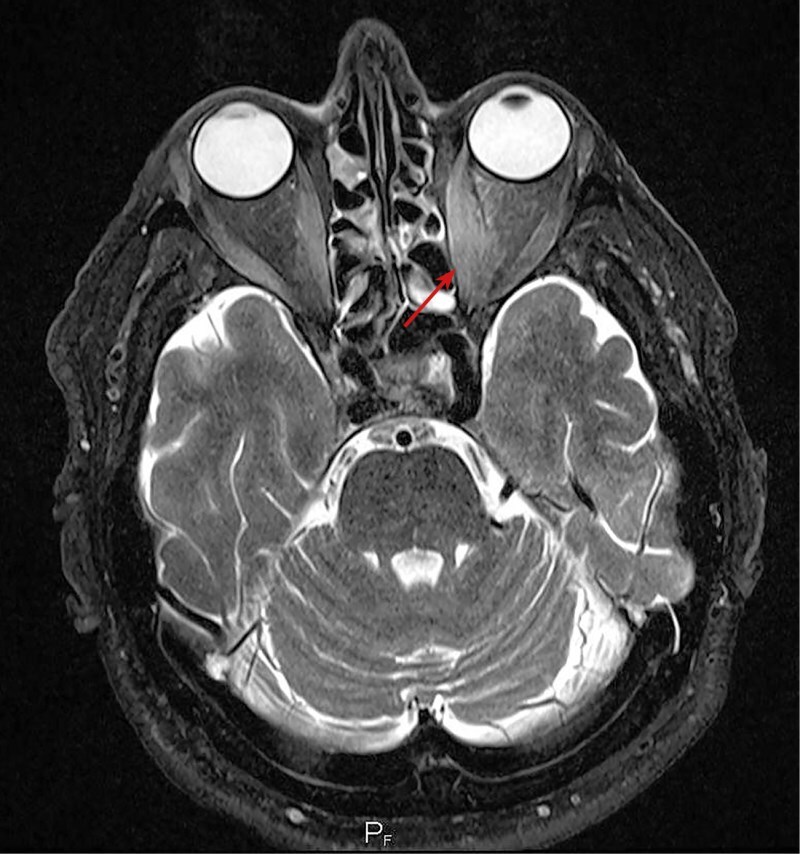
Figure 2. MRI scan showing muscle enlargement without compressing the optic nerve.
